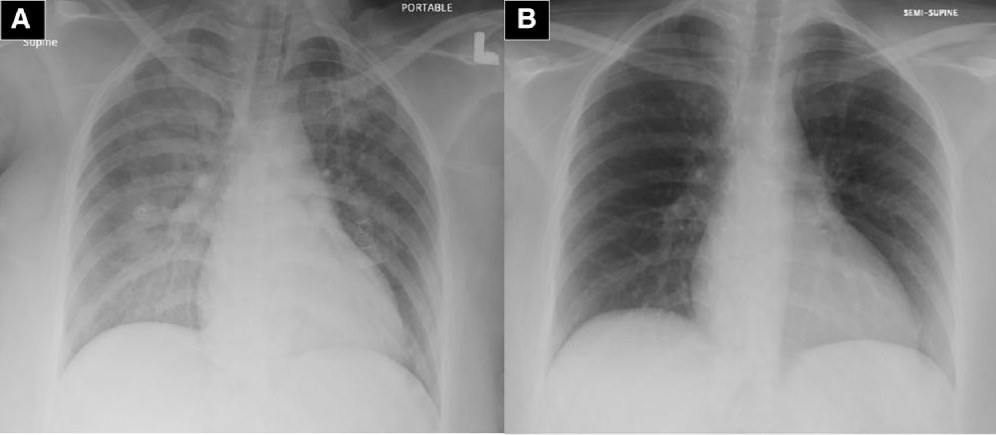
(*A*) Initial chest x-ray on day of admission showing bilateral lung congestion and features of pulmonary oedema. (*B*) A chest x-ray on Day 2 of admission with marked improvement and disappearance of pulmonary congestion.
